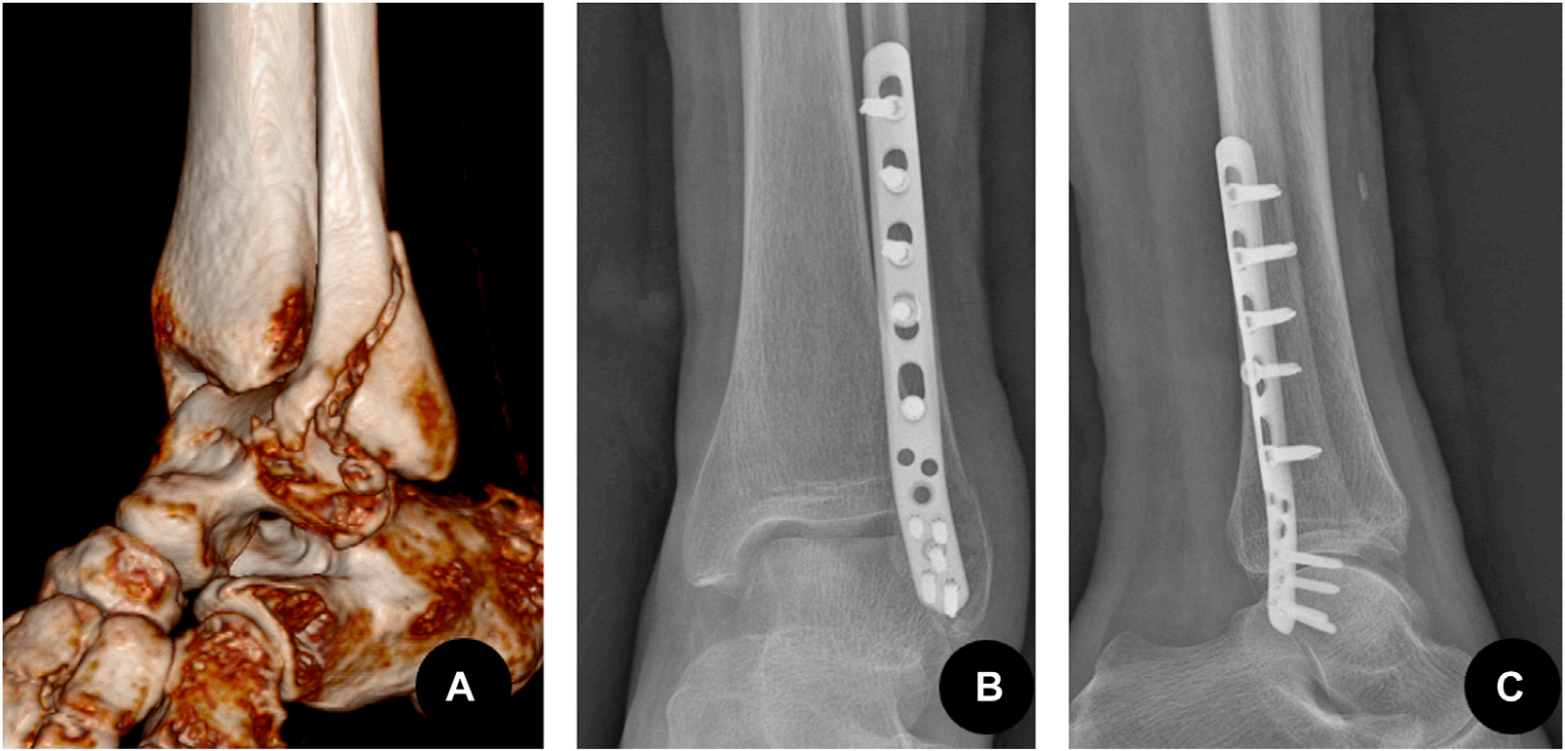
Type B lateral malleolar fracture fixed by the antiglide-buttress plate mode that is applied on the posterolateral side. (A) A 72-year-old female patient with injury to the left lateral malleolus. Preoperative CT three-dimensional reconstruction showed a type B spiral fracture, the distal fragment moved backward and upward. (B) The postoperative anteroposterior X-ray showed that the fracture of the lateral malleolus was fixed by the posterolateral anti-glide plate. (C) Postoperative lateral X-ray.
